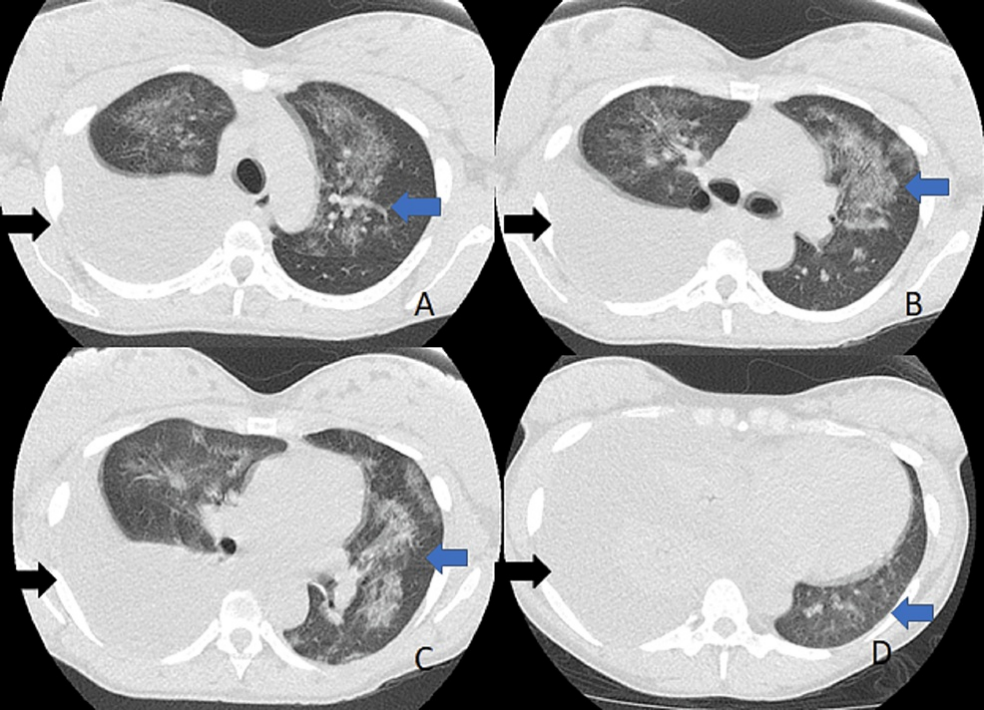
Initial CT of the chest and lung windows Right pleural effusion (black arrows) and pulmonary infiltrates (blue arrows). A. upper lobes; B. carina; C. middle lobe; D. lower lobes
